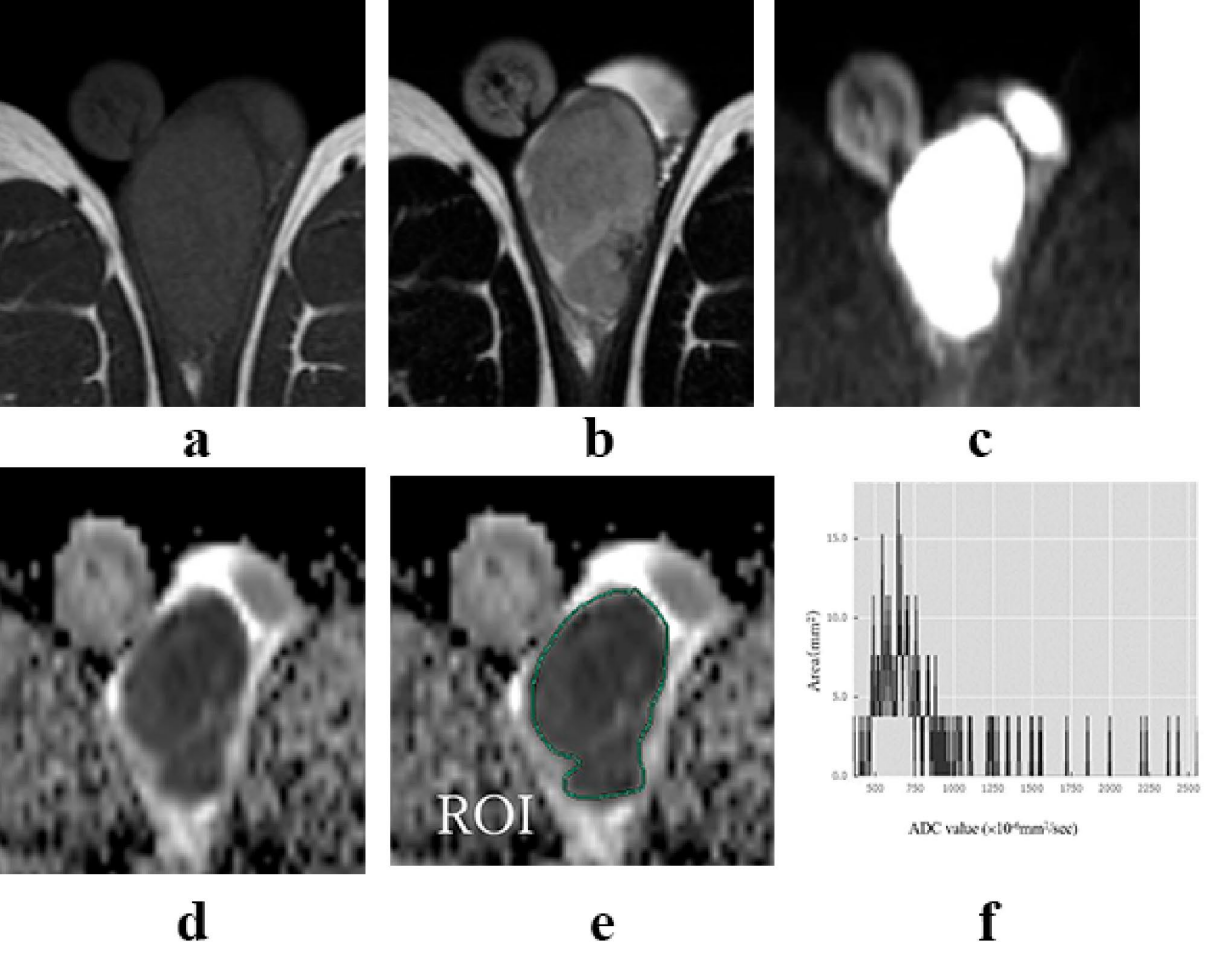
Representative findings of seminoma. T1W1 (a), T2W1 (b), DWI (c), and ADC map (d). The ROI surrounded the entire tumor on the ADC map (e). Histogram of ADC value (f). The histogram of the seminoma showed high kurtosis and skewness values of 15.318 and 3.21, respectively. It showed the distribution with peaked and heavy fringes compared with the normal distribution, and the mean ADC values were skewed toward the lower end of the distribution. T1WI: T1-weighted imaging, T2WI: T2-weighted imaging, ADC: apparent diffusion coefficient, ROI: region of interest, DWI: diffusion-weighted imaging
